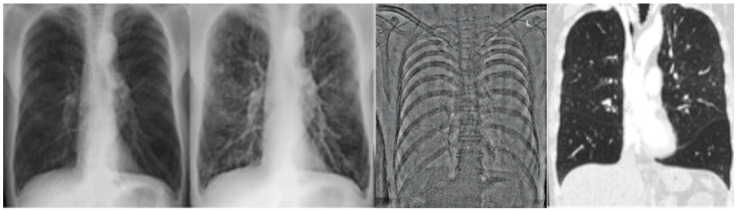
Moderate emphysema, right upper lobe and left lower lobe. Left: conventional x-ray postero anterior (p.a.), middle: dual energy x-ray p.a., right: corresponding computed tomography image.
